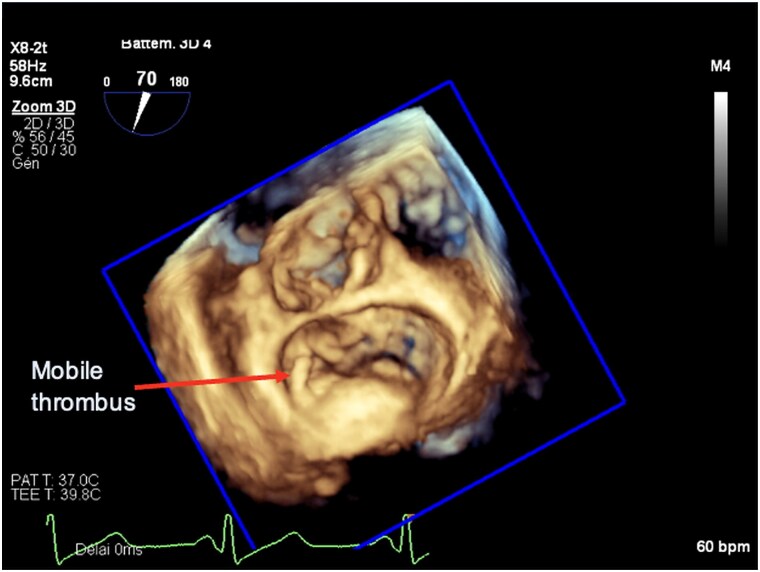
Transoesophageal three-dimensional echocardiography (3D surgical view) showing the mass arising from the posterior mitral annulus with mobile thrombus attached.
